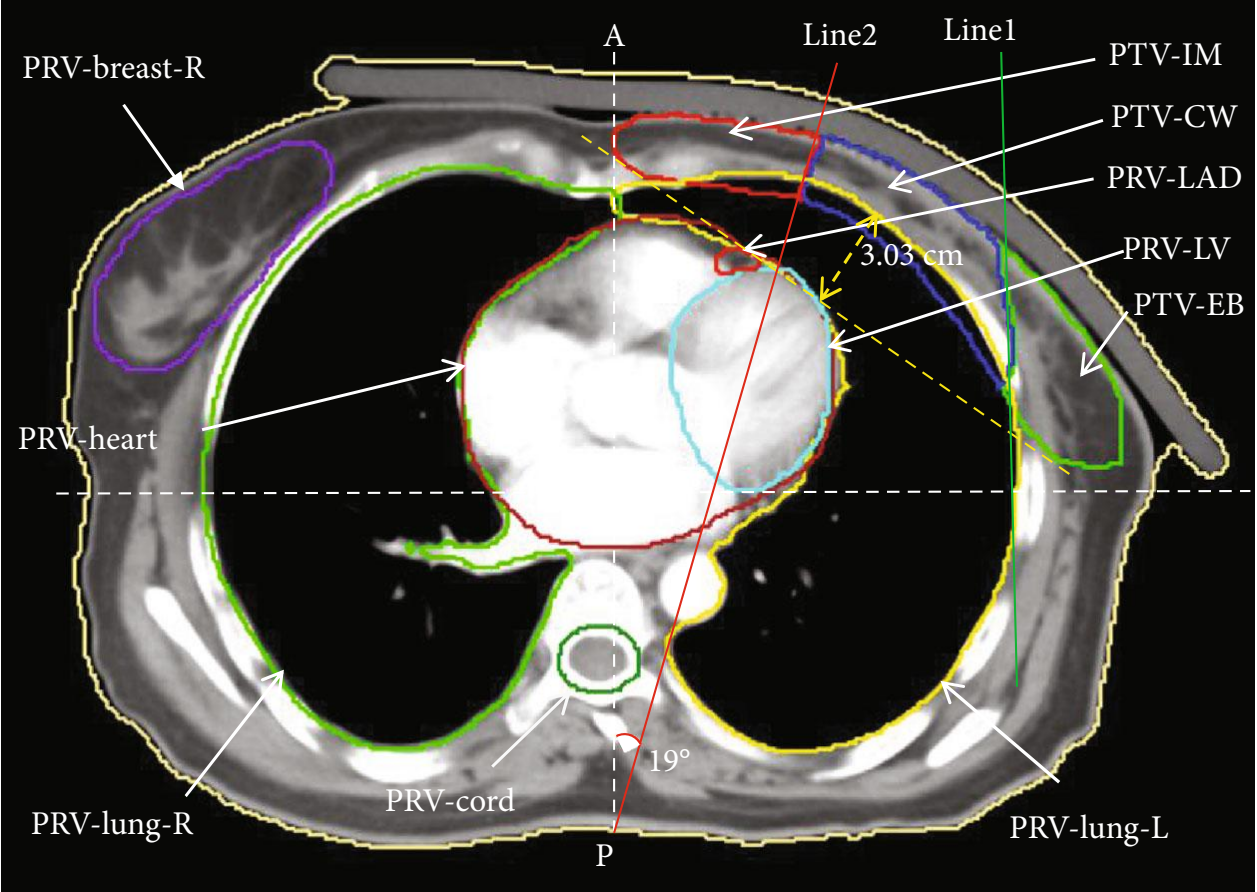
Targets segmental setting of TSP in an axial view of CT. Abbreviations: PTV-CW: chest wall region of PTV (blue); PTV-IM: internal mammary nodes region of PTV (red); PTV-EB: external breast region of PTV (green).
